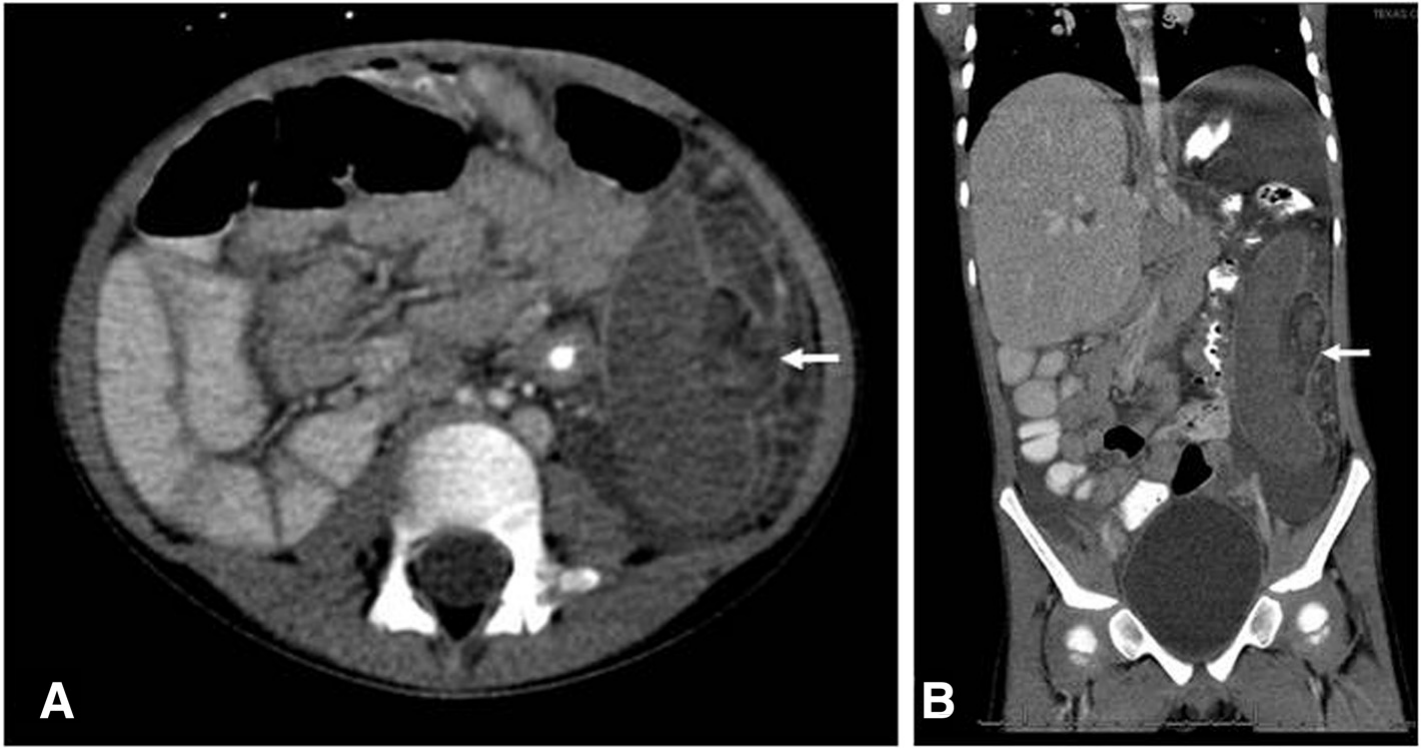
CT imaging of infarcted spleen: (A)Axial image showing global infarction.(B) Coronal view of CT scan of abdomen and pelvis with enteral and intravenous contrast. There is lack of contrast enhancement of the spleen with the hilum rotated externally (arrow).
